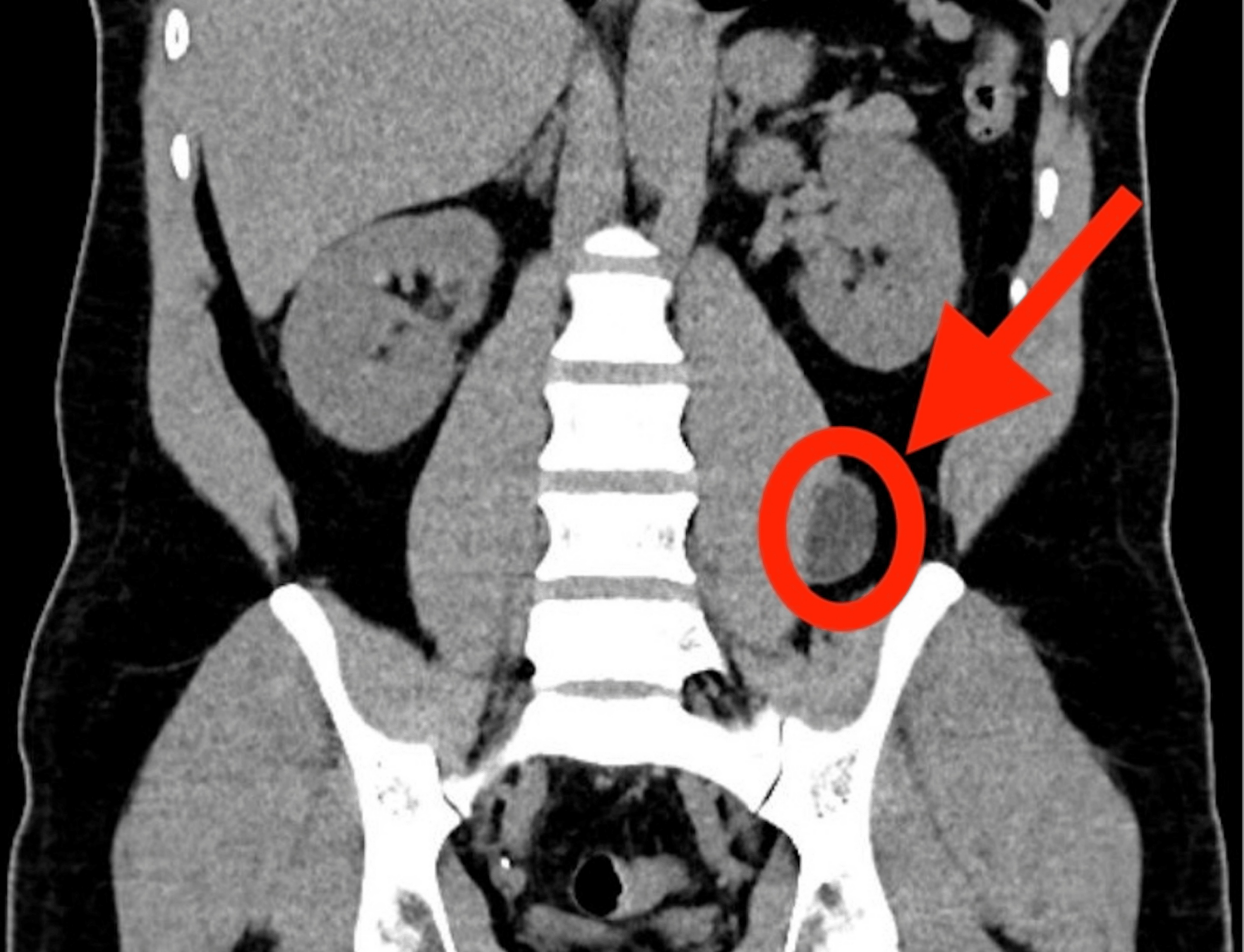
Coronal CT of the patient showing psoas abscess (red circle and arrow) shown on the lateral aspect of the left psoas muscle CT: computed tomography
